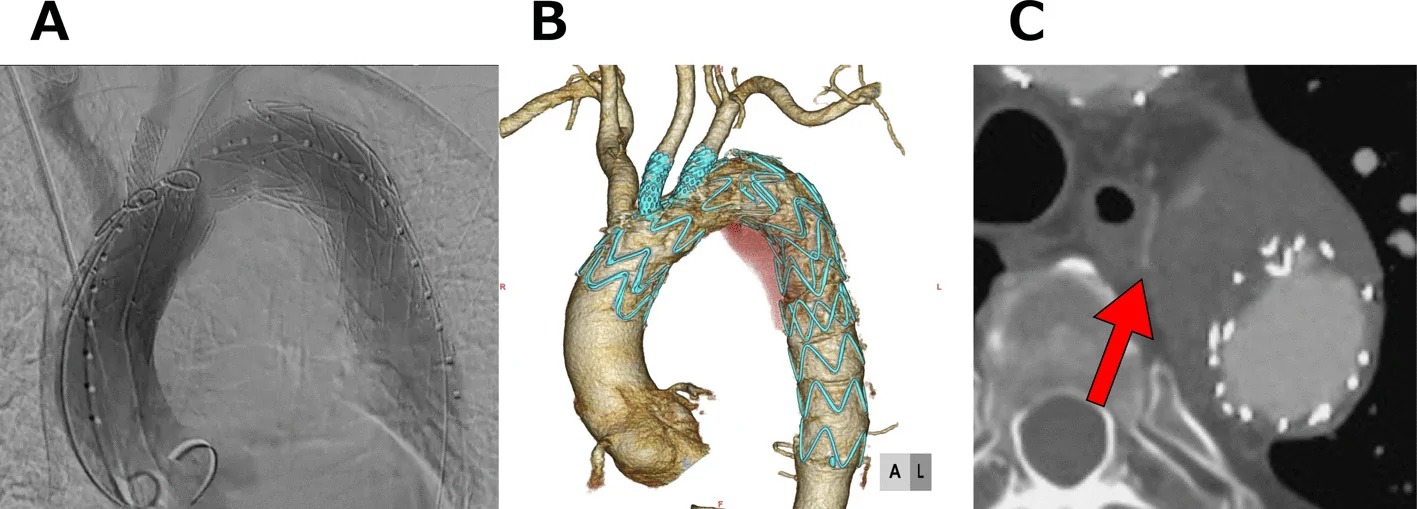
A Final intraoperative angiography. No obvious endoleak was observed. B 3D CT obtained on postoperative day 3. The type Ia endoleak had disappeared, and the patency of the aortic arch branches was good. Although a type II endoleak was observed, no findings requiring reintervention were identified. C Axial contrast-enhanced arterial-phase CT obtained on postoperative day 3. The bronchial arteries were enhanced as an inflow artery of a type II endoleak. The outflow arteries were not clearly visualized. 3D: 3-dimensional; CT: computed tomography
